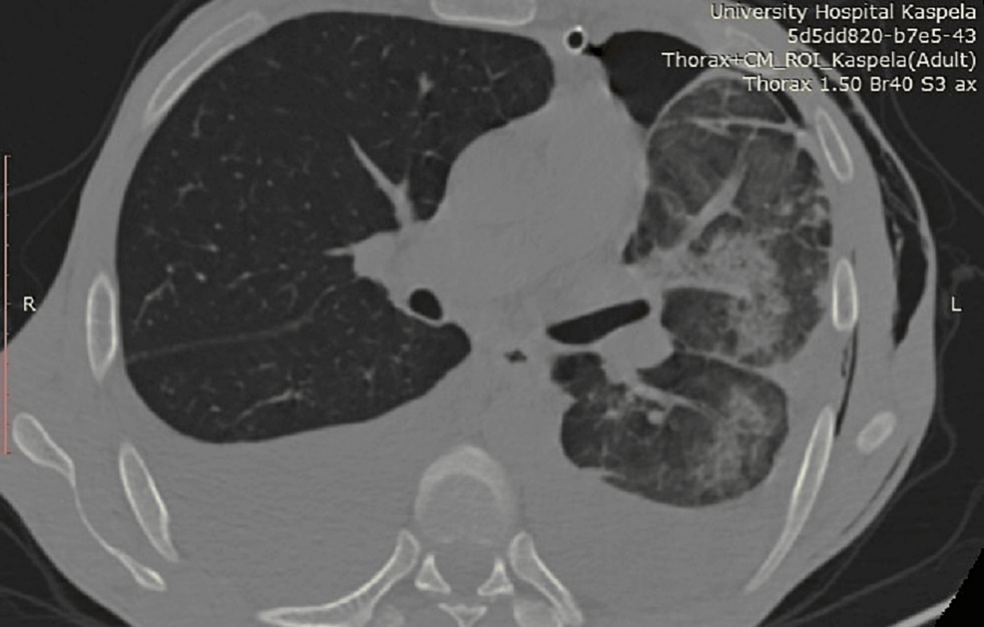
An axial view of the CT just after a thoracostomy tube is placed, noting the bilateral effusions
